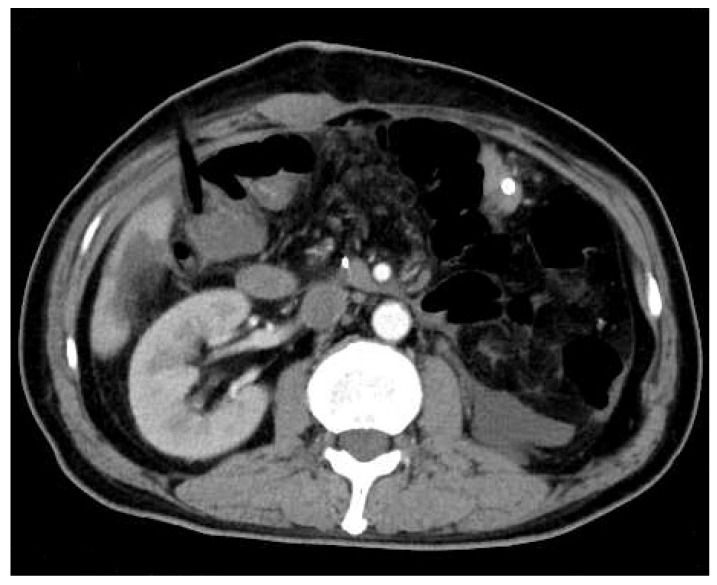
Abdominal enhanced computed tomography (CT) was rechecked on the fourth postoperative day. It showed no obvious effusion or residual lesion in the abdominal cavity.
